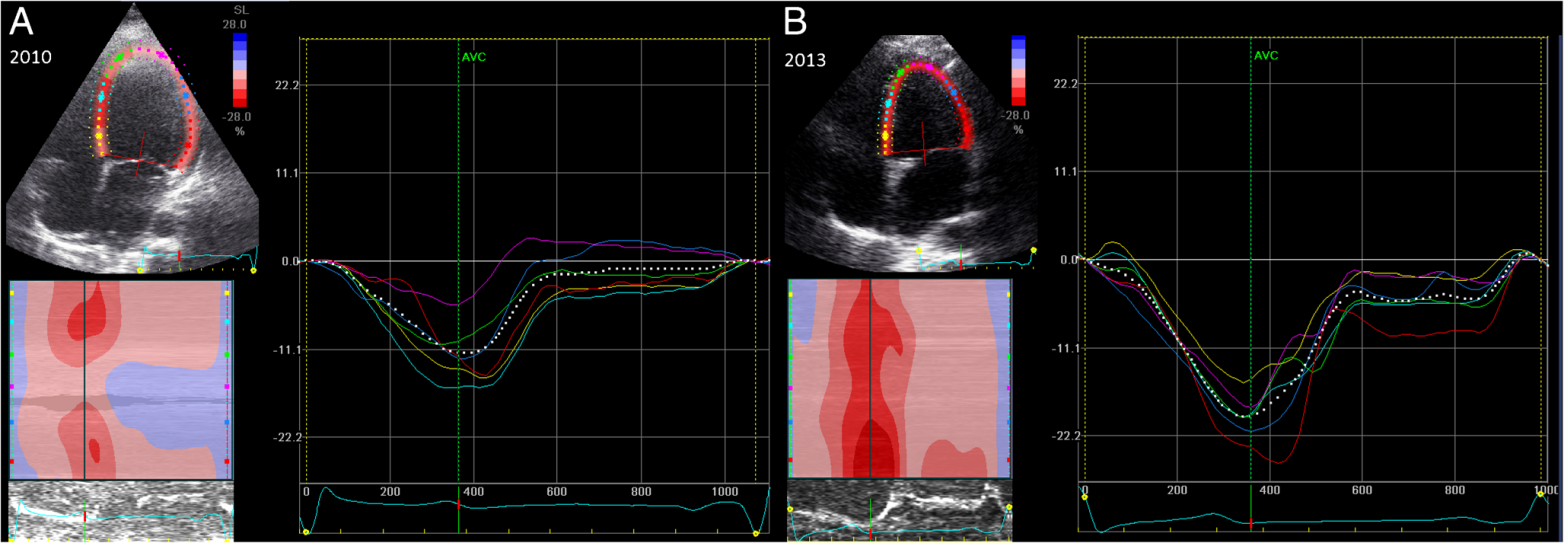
Two-dimensional strain measurement. Two-dimensional strain measurement in longitudinal plane. At the left top, the region of interest is shown, at the right the graphic depicture of the transmural strain in a young female m.3243A>G carrier who was found to have severe cardiomyopathy in 2010. After diagnosis, she was started on medication (diuretics, ACE inhibitor and β blocker) and underwent an intensive heart failure rehabilitation programme. At the echocardiogram in 2013, she reported a highly significant increase in exercise tolerance. The coloured lines represent the measurements of regional deformation of the individual regions, the dotted line represents their average (global strain), analysed by GE EchoPac. GLS increased from −12.7 to −19.6
